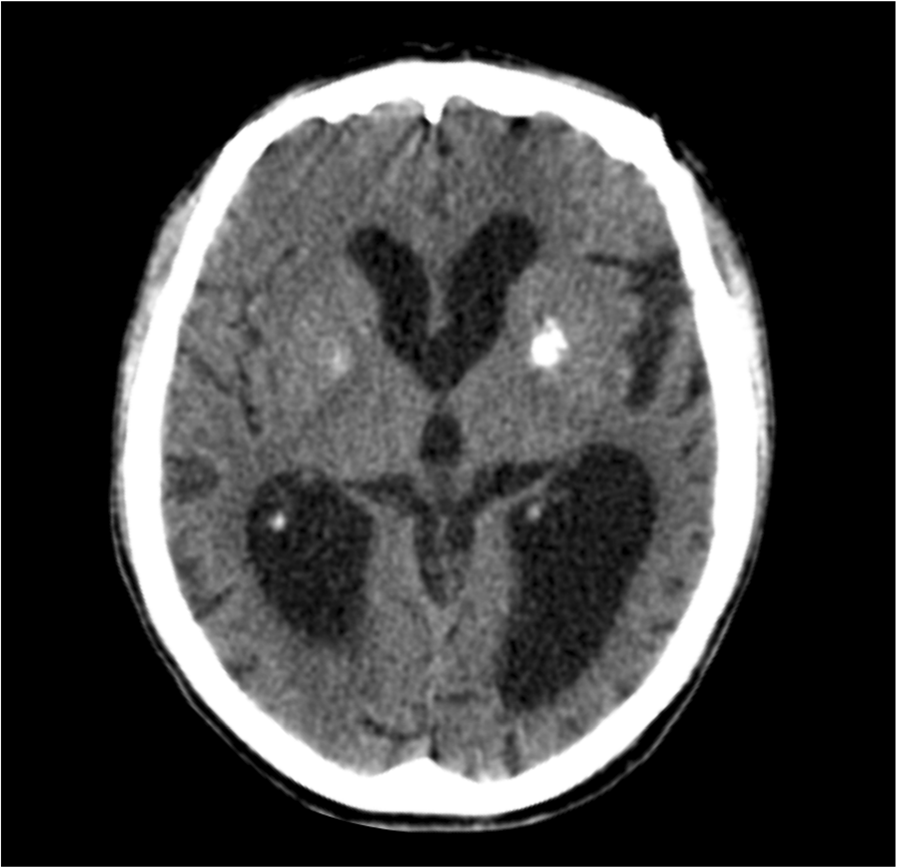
Axial CT of a 46-year-old Down syndrome patient with basal ganglia calcifications, mainly involving the *globus pallidus*
